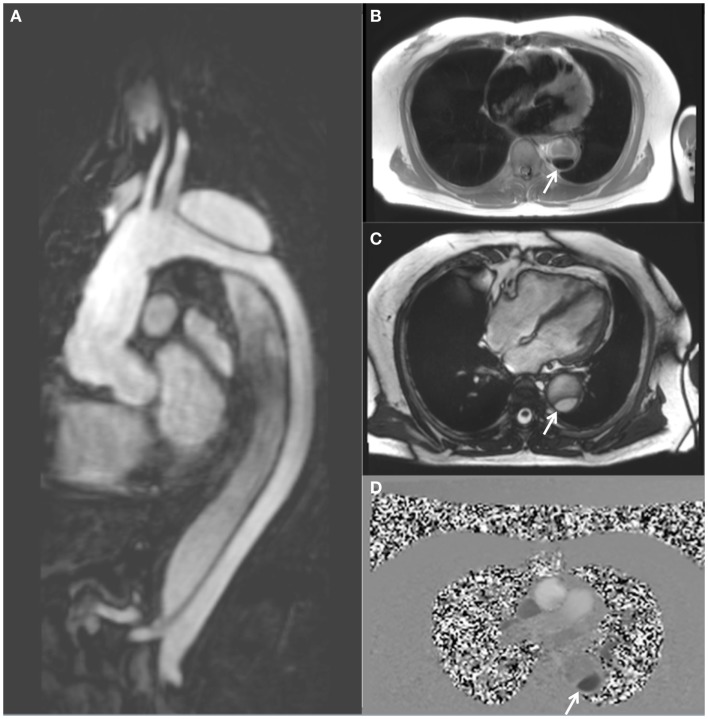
MRI follow-up of a 63-year-old male with chronic descending thoracic aortic dissection. The patient had undergone surgical replacement of the ascending aorta for type A aortic dissection 4 years earlier. (A) Sagittal gadolinium-contrast-enhanced MRA (magnetic resonance angiography) view; (B) axial black blood view of the proximal descending thoracic aorta; (C) axial true FISP (steady state-free precession) cine view; and (D) axial phase-contrast view, showing flow patterns in the true and false lumens of the descending aorta. The true lumen is indicated by the white arrow (Courtesy: Cardiovascular Magnetic Resonance, Sydney, Australia).
